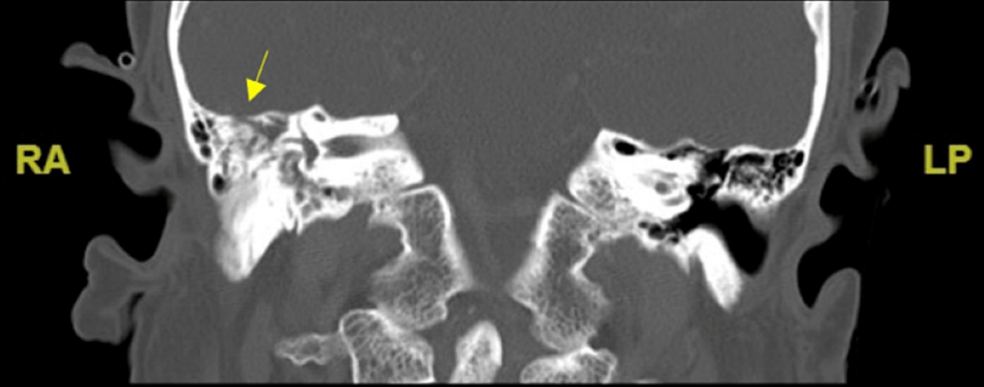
CT evaluation of the internal auditory canal, posterior fossa, and maxillofacial area showing a right tegmen tympani defect and serous effusion, consistent with AOM. AOM: acute otitis media
